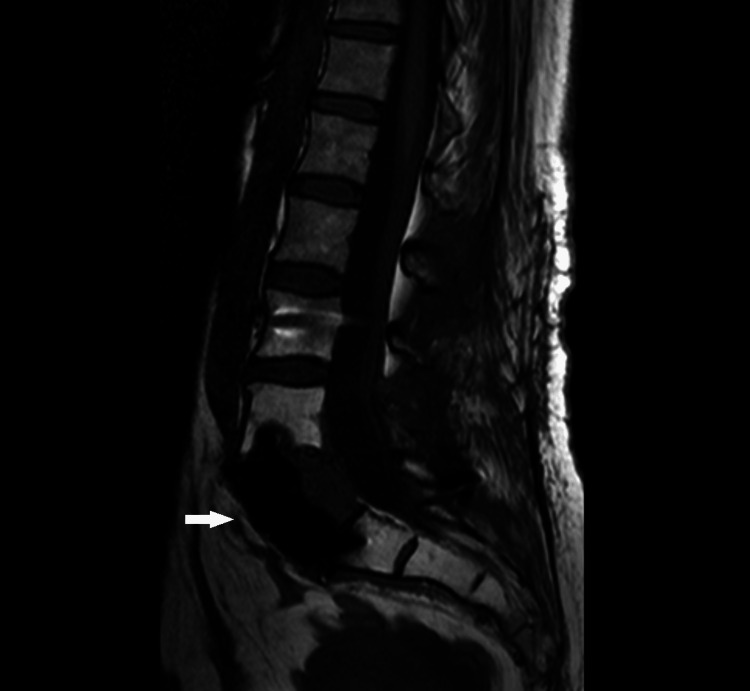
Lumbar MRI, sagittal T1WI sequence. Lumbar MRI after surgery showed no persistence of disease on sagittal T1WI.
